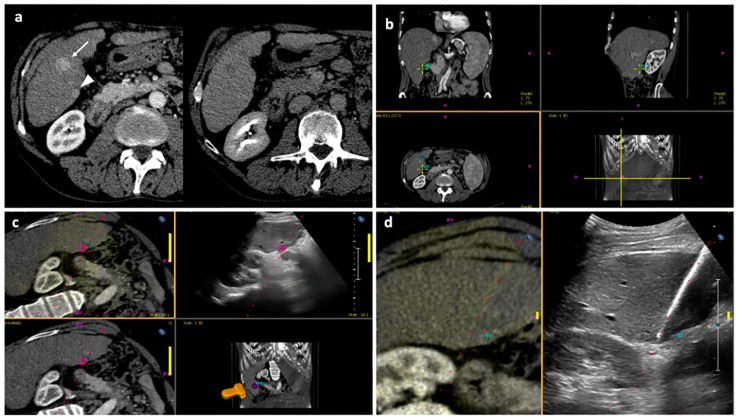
“Double fusion” technique—Part 1. (a) Pre-procedural axial CT images in the arterial and delayed phase showing a larger nodule with wash-in and wash-out consistent with HCC in segment V (white arrow), which was visible at US examination, and a smaller posterior subcapsular nodule with the same features (white arrowhead), which was not visible at US. The patient was scheduled for percutaneous MWA of the larger nodule under US guidance and of the smaller nodule with US fusion imaging guidance. (b) The smaller tumor is selected on the US scanner by visualization of pre-procedural CT images in the axial, coronal and sagittal planes. (c) Once registration of pre-procedural CT images to real-time US images are performed, the fused images are shown at the same time on the left upper quadrant of the US screen, simple US images are displayed on the right upper quadrant, CT images are shown on the left lower quadrant and the US probe 3D position is shown on the right lower quadrant. In this image, the smaller target tumor is visualized as a pink circle. (d) When US fusion imaging quality is judged adequate, the MWA antenna is placed into the target tumor; fused images are shown on the left while simple US images are shown on the right. Abbreviations—CBCT: cone-beam computed tomography; CT: computed tomography; MWA: microwave ablation; US: ultrasound.
